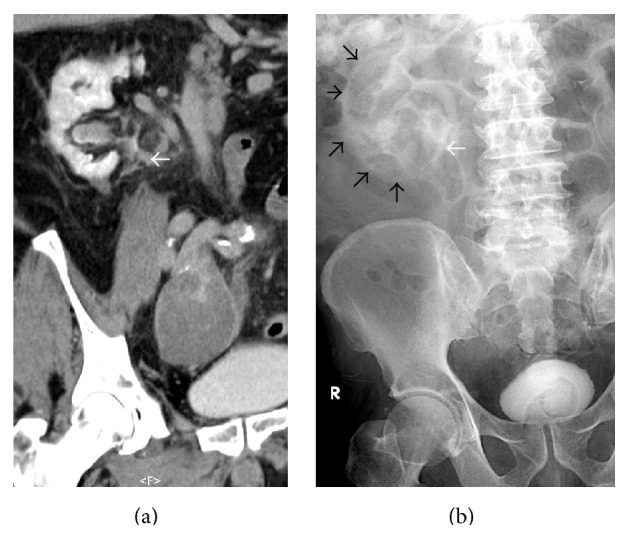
(a) The multiplanar reformation of the CT in excretory phase of the abdomen shows a collection of excreted contrast mixed with urine (arrow) in the soft tissue at renal hilar and parahilar regions. (b) The plain KUB radiograph after CT scan shows the correlated area of contrast leakage (white arrow) seen from CT scan and also additional perinephric contrast collection (black arrows).
